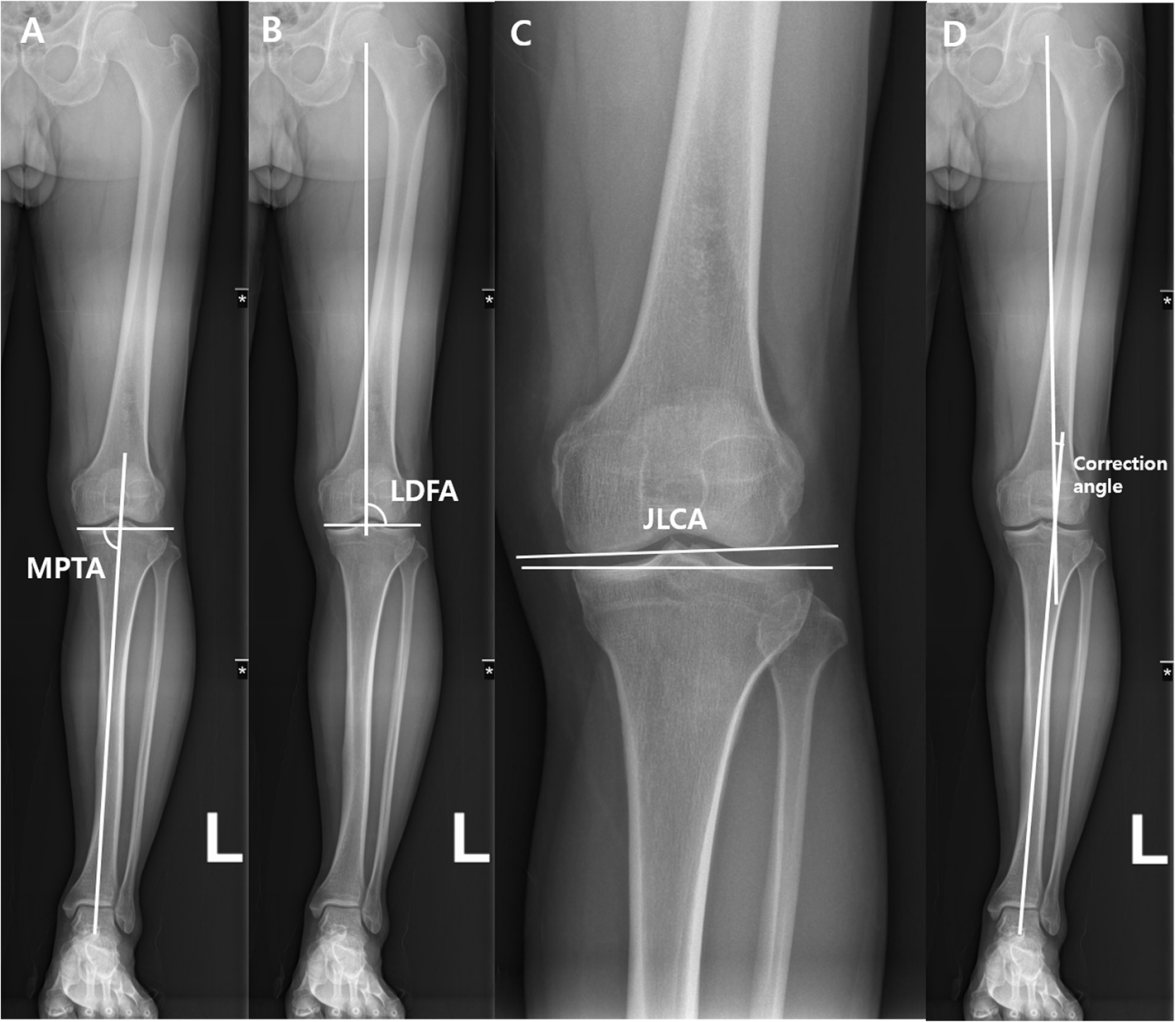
Radiographic parameters for evaluation of coronal alignment and correction angle of the knee using whole leg anteroposterior radiographs. Medial proximal tibial angle (MPTA) (A), lateral distal femoral angle (LDFA) (B), joint line convergence angle (JLCA) (C), correction angle as planned by Dugdale method (D)
